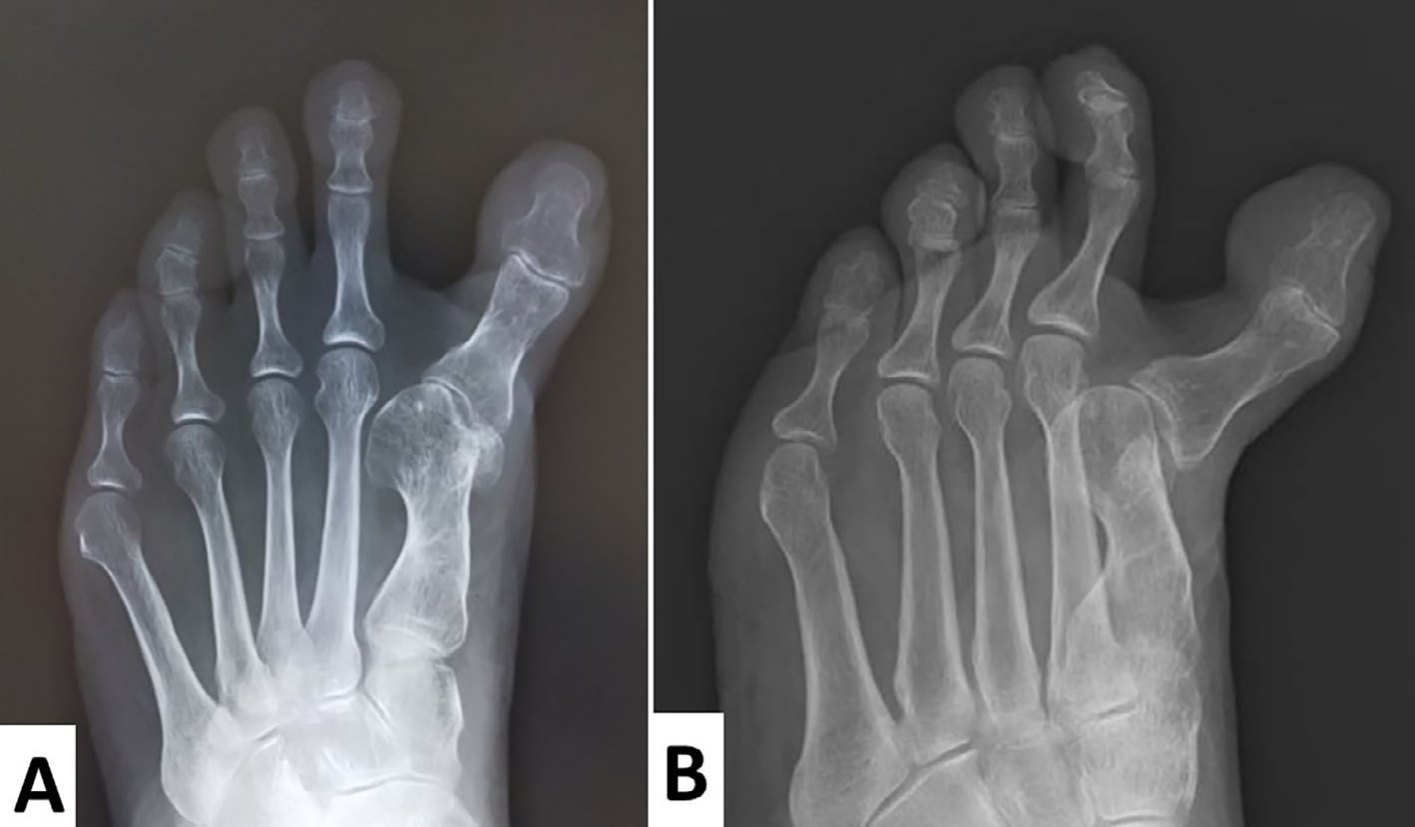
Passive motion of metatarsophalangeal (MTP) and interphalangeal (IP) joints of the left hallucis. Radiographs show that the hallux valgus angle was -28°. The intermetatarsal angle, which should be approximately 6°–9°, is 0°. This indicates that the first and second metatarsal bones are parallel. The tibial sesamoid has shifted medially and the fibular sesamoid is absent. Excessive medial eminence resection might have been performed.
